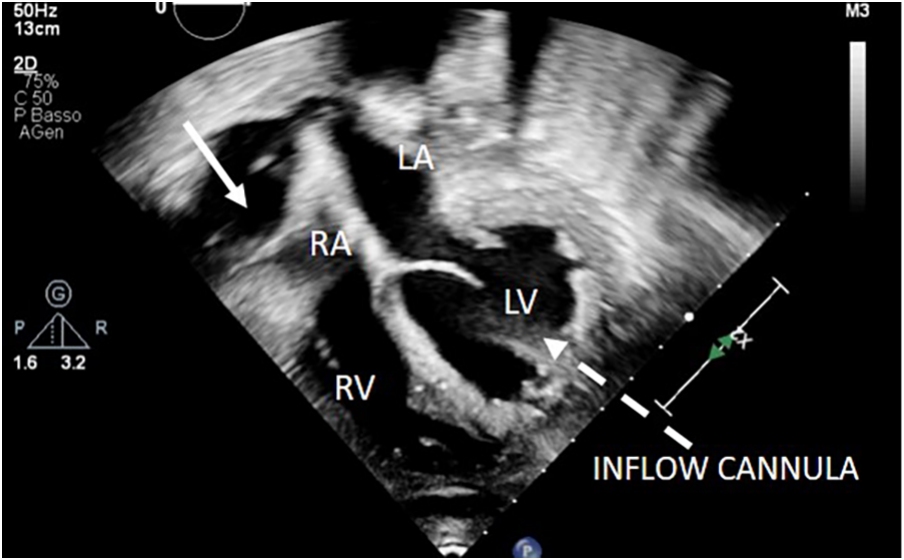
2D apical 4C view showing right atrium collapse due to retroatrial effusion after LVAD implantation. LVAD inflow cannula: dotted arrow. Retroatrial effusion: arrow.
